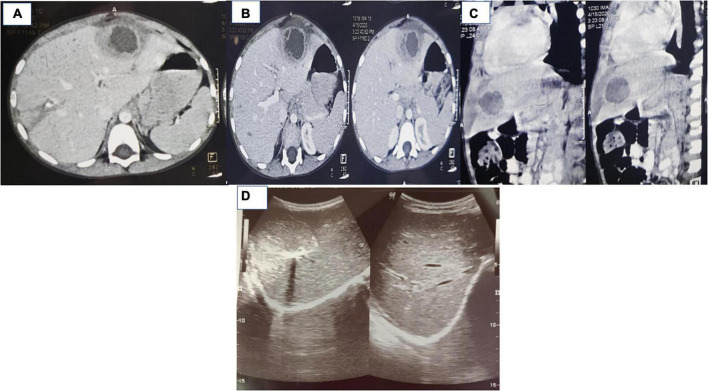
(A–C) Computed tomography of case 2 showing a hypoattenuating focal lesion with thick/shaggy walls with perilesional edema suggestive of abscess in the liver, arrow marks. (D) Ultrasound images of case 2 showing resolution of abscess following initiation of corticosteroids (after 6 weeks of initiation of therapy).
